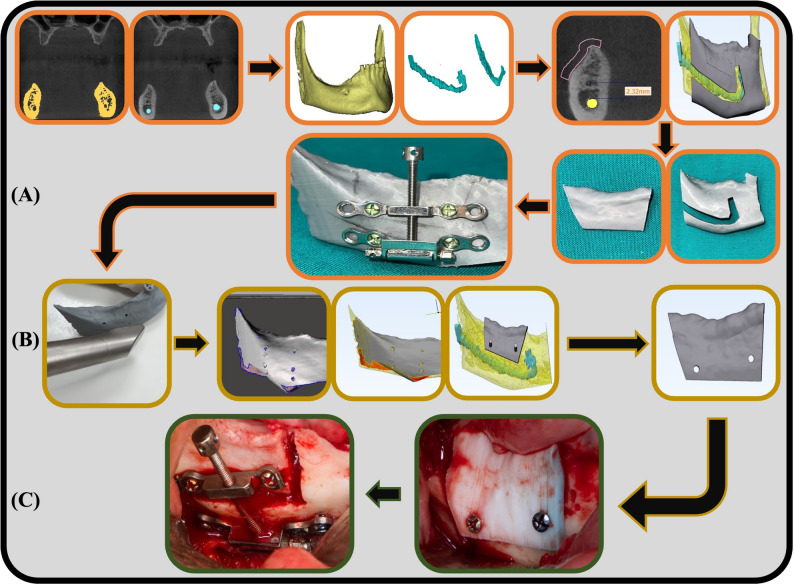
Double Scan Virtual planning protocol for application of Bi-Axial extraosseous alveolar distraction device in vertically deficient posterior mandibular region. The first radiographic scanning part of the virtual plan is presented in segment (A), which consists of mandible and IAN thresholding and 3d-model creation, IAN outlining and provisional osteotomy guides designing and 3D-printing, nerve and osteotomy outlining, and provisional distractor fixation. The second optical scanning part is presented in segment (B), which consists of model scanning, superimposition, final guide designing, and osteotomy cutting guide printing. Segment (C) shows osteotomy cutting guide intraoperative fixation and device installation
